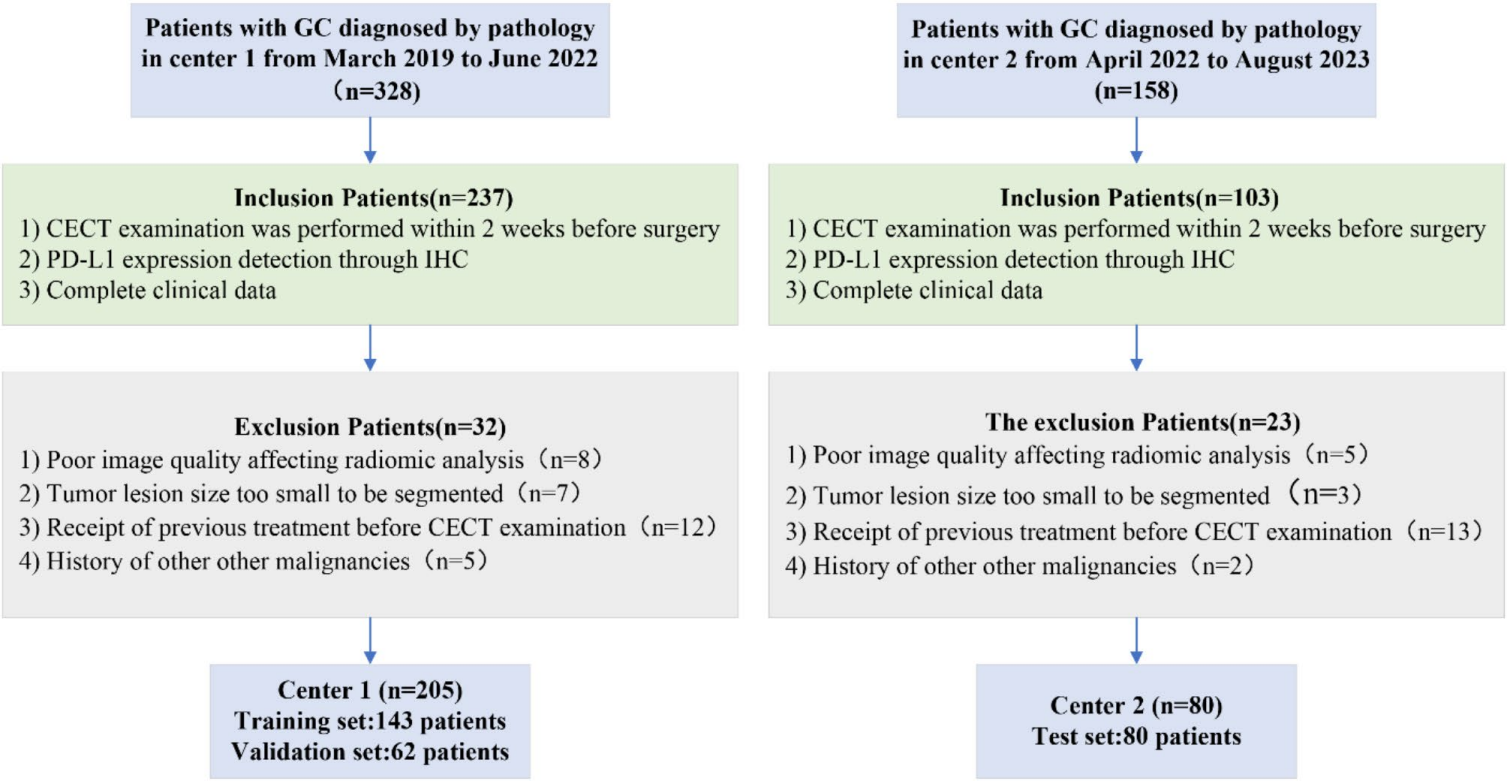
Flow of patient recruitment. PD-L1, programmed death-ligand 1; CECT, contrast-enhanced computed tomography
